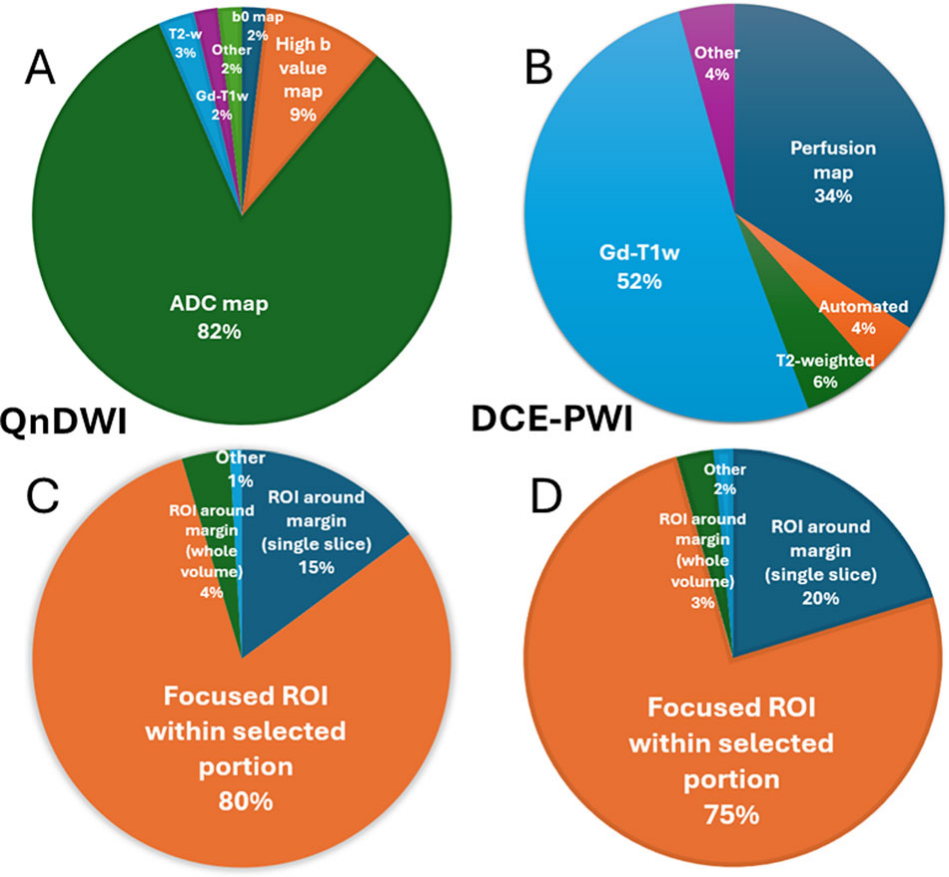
Pie charts demonstrating the sequences primarily used to draw ROIs for the analysis of (A) Qn-DWI (*n* = 108) and (B) DCE-PWI (*n* = 70) and the methods of ROI placement for (C) QnDWI (*n* = 108) and (D) DCE-PWI (*n* = 70). The percentage of total responses to the question is indicated
